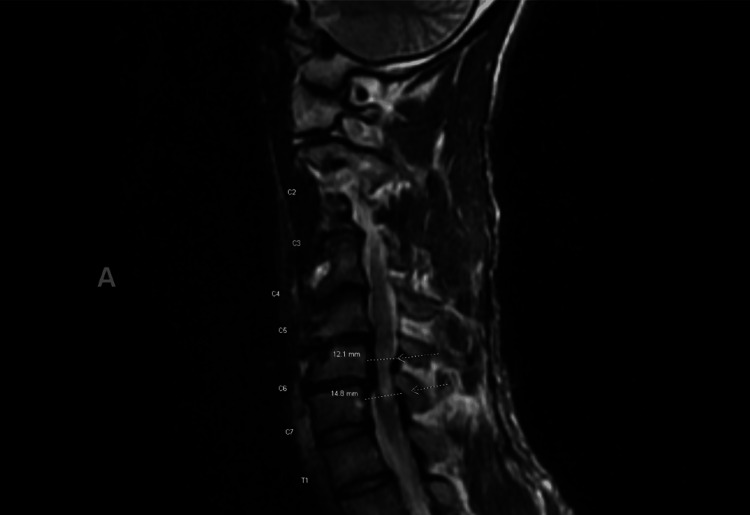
Cervical spine showing myelopathy and myelomalacia from severe spinal canal stenosis greatest at C6-C7 with chronic cord flattening from retrolisthesis and endplate osteophytes and ligamentum flavum buckling. T2-weighted sagittal view of MRI. Arrows indicate the extent of severity of canal stenosis with flattening of the cord.
